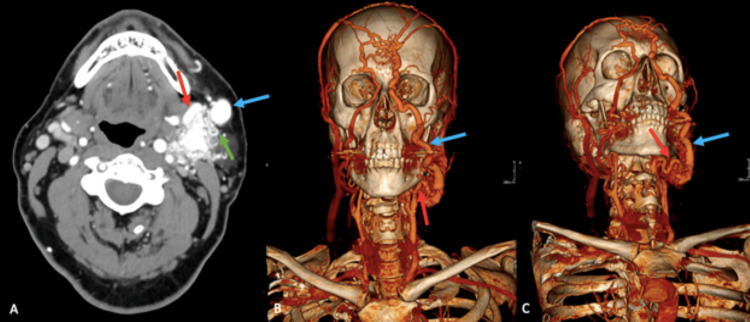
Head and neck computed tomography angiography. The computed tomography angiography images (A, B, C) with volume rendering reconstruction show a large CAVF from the left facial artery (red arrows) to the left facial vein (blue arrows). Notice the prominent abnormal blood vessels, most likely consisting with congested draining veins (green arrow). The middle third of the left external jugular vein is not seen due to ligation during a previous surgical procedure to remove a left carotid paraganglioma. CVAF: cervical arteriovenous fistula
